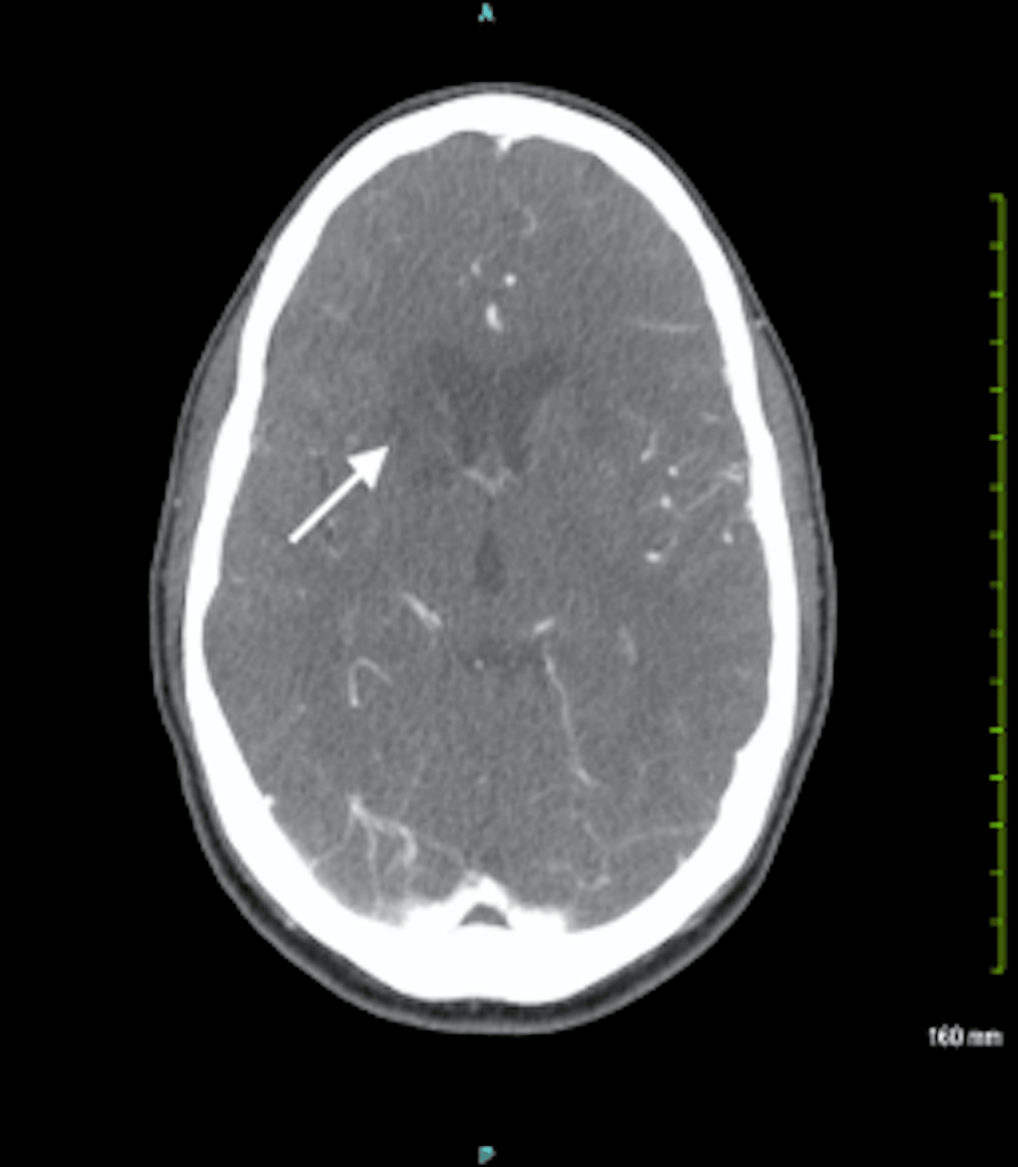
CT imaging of the head, demonstrating a right basal ganglia infarct (arrow), resulting in contralateral (left-sided) neurologic manifestations
